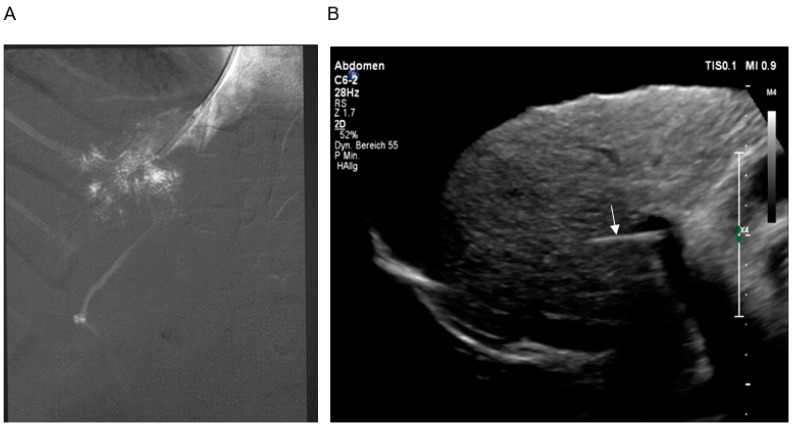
(A) Hand-injection of contrast into the parenchyma of the liver showing retrograde filling of a portal branch. (B) Sonographic guidance of the puncture. The location of the needle (arrow) and the portal vein is demonstrated.
